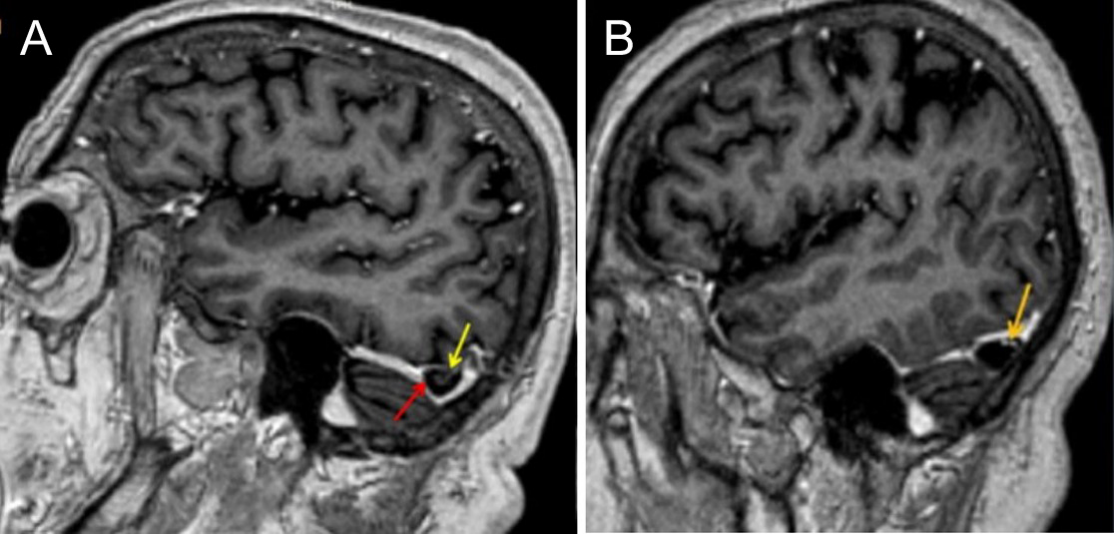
A, B. Sagittal post-contrast T1-weighted MR images show brain herniation (yellow arrow) into an intra-sinus giant arachnoid granulation (red arrow) in the midlateral of the right transverse sinus. MR, magnetic resonance.
